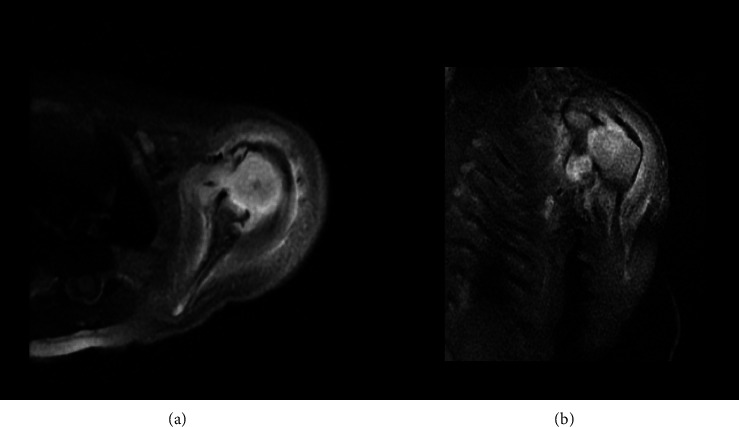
Axial (a) and coronal (b) sections of MRI (*T2-weighted*) images demonstrating left glenohumeral joint effusion and synovial thickening suggestive of synovitis, in keeping with septic arthritis.
